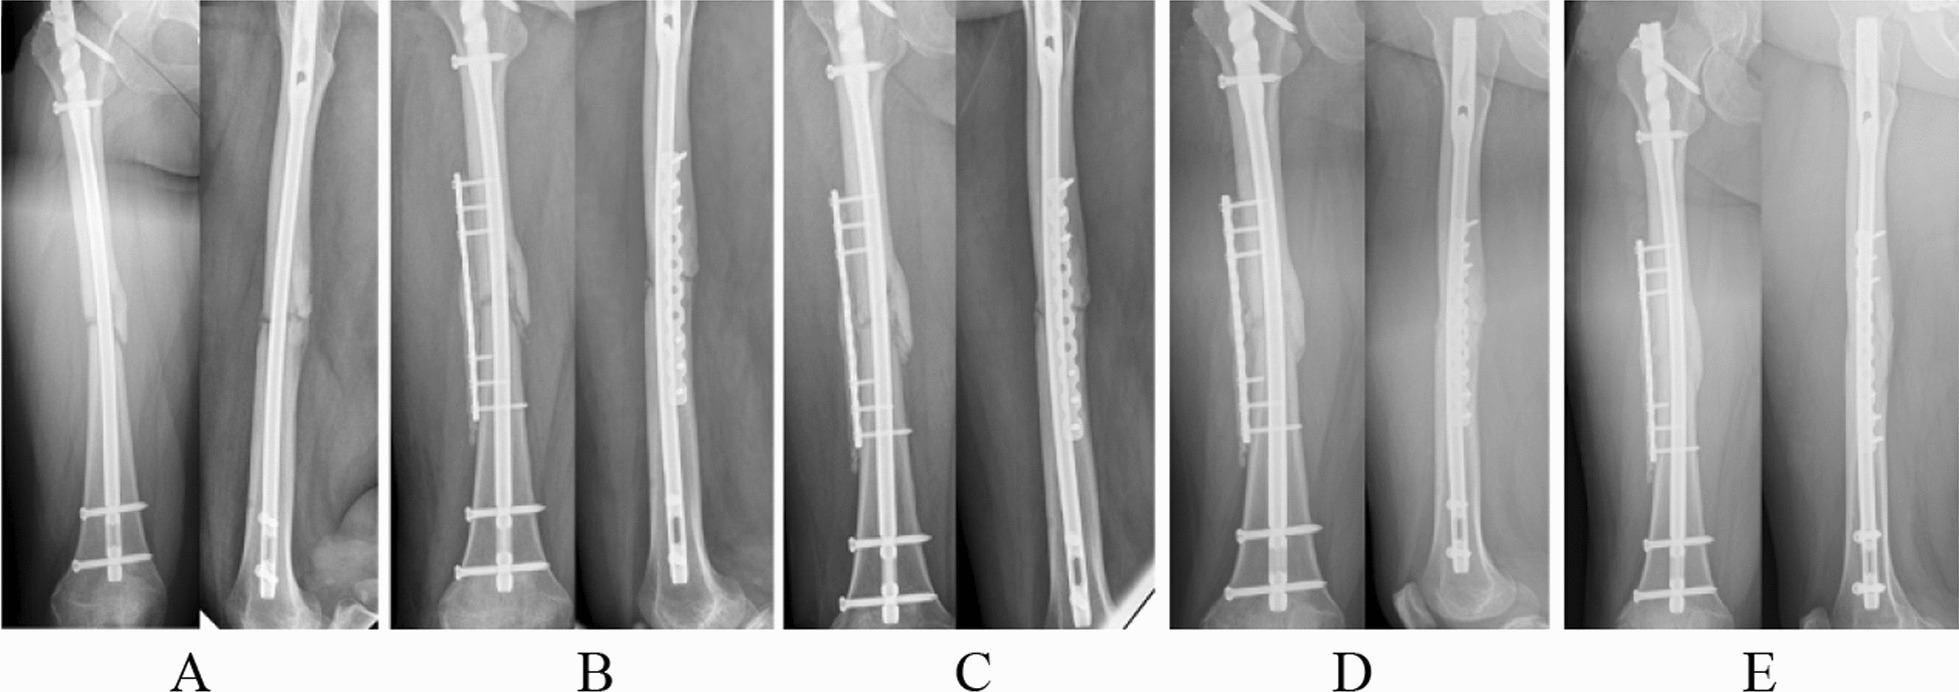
A female patient (25 years old) with bone nonunion in the right femur was treated with one-stage autologous bone grafting and had an open fracture before; no bacteria were detected after surgery. A Anteroposterior and lateral radiographs showed a clear fracture line and less callus formation at the fracture site after 12 months of initial osteosynthesis; B–E radiographs at 2, 6, 12, and 18 months after one-stage autologous bone grafting treatment. Bone union was achieved at 12 months, and complete bone consolidation was achieved at 18 months
